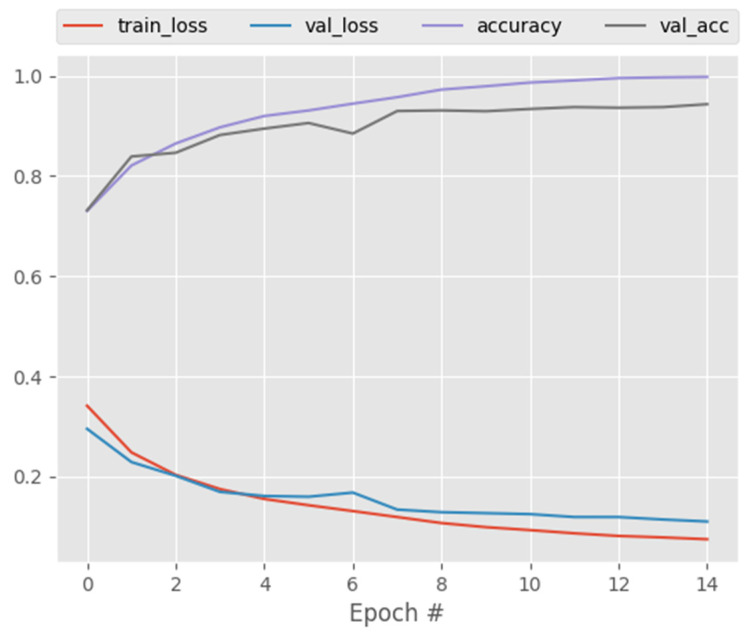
CapsNet training and validation performance for X-ray images.
